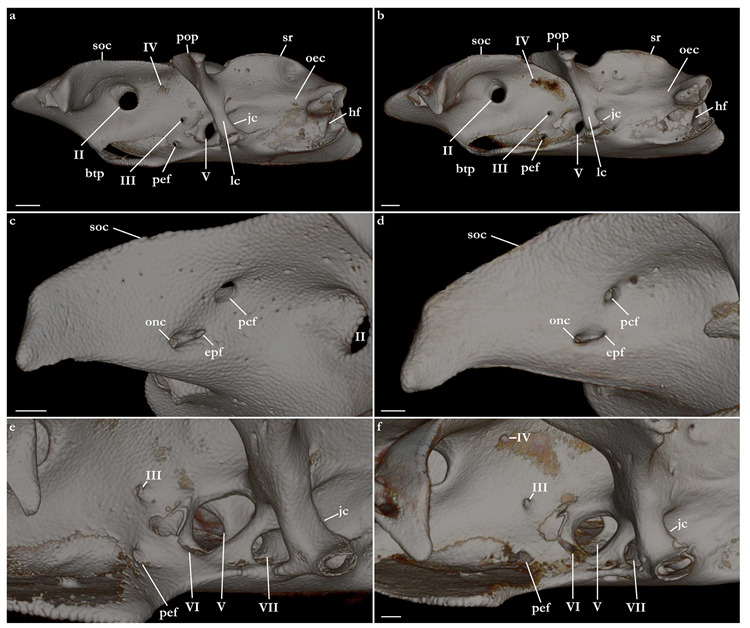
Micro-computed tomography scans of the neurocranium of (a,c,e) *Squatina leae* sp. nov., paratype, ZMH 26098, juvenile male, 249.6 mm TL and (b,d,f) *S. africana*, ZMH 123064, juvenile female, 309 mm TL in lateral view. Abbreviations: btp, basitrabecular process; epf, external profundus foramen; hf, hyomandibular facet; jc, jugular canal; lc, lateral commissure; oec, otic external canal; onc, orbitonasal canal; pcf, preorbital canal foramen; pef, pseudobranchial artery foramen; pop, postorbital process; soc, supraorbital crest; sr, sphenopterotic ridge; II, optic nerve foramen; III, oculomotor nerve foramen; IV, trochlear nerve foramen; V, trigeminal nerve foramen; VI, abducens nerve foramen; VII, facialis nerve foramen. Facing left; scale bars (a,b): 2 mm, (c,d,f): 1 mm.
